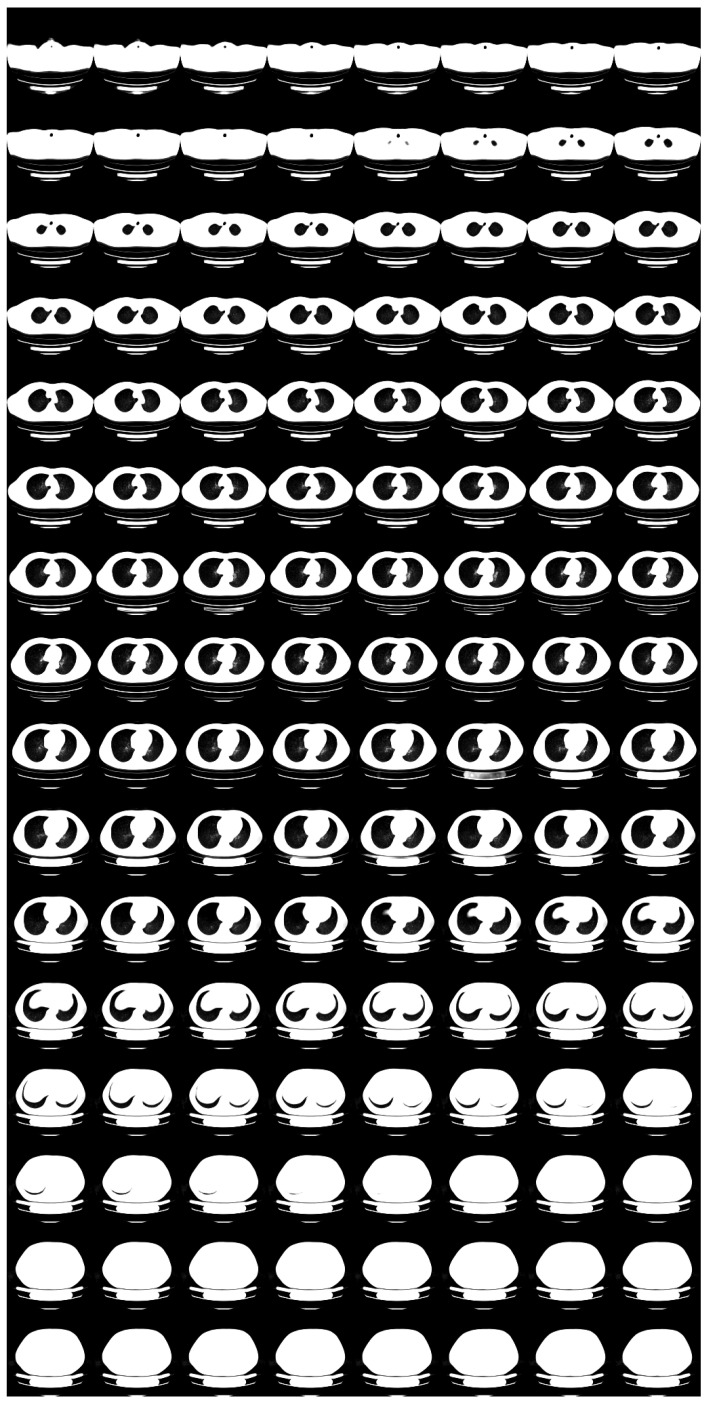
Reconstructed 3D CT image with X2CT-FLOW from Figure 7c,d ($\sigma^{2}=100,N=2$), in a pulmonary window setting.
